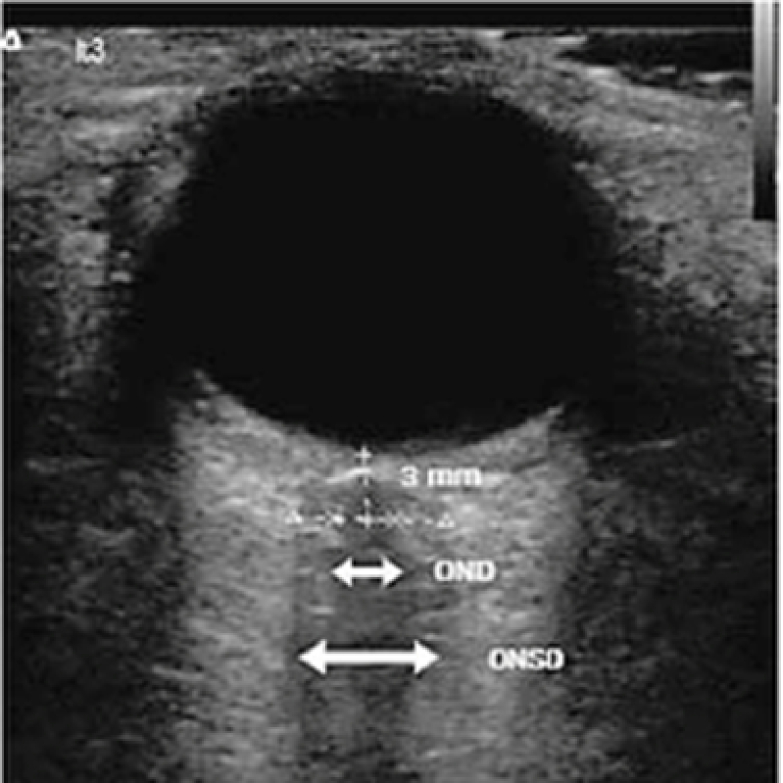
Ultrasound of the eye depicting anatomical landmarks. Optic Nerve Diameter (OND) and Optic Nerve Sheath Diameter (ONSD) were measured 3 mm from the back of the globe in this study.^[19]^.
